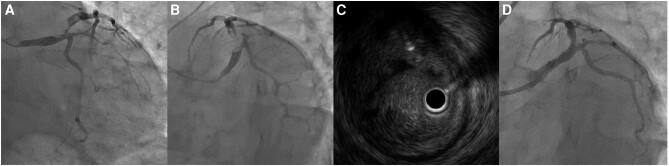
In coronary angiography at presentation, AP caudal (*A*) and LAO caudal (*B*) views showed a lesion from left main trunk to left anterior descending artery. Intravascular ultrasound showed massive thrombus (*C*). LAO caudal view after excimer laser coronary angioplasty revealed improvement of coronary stenosis (*D*). AP, anteroposterior; LAO, left anterior oblique.
